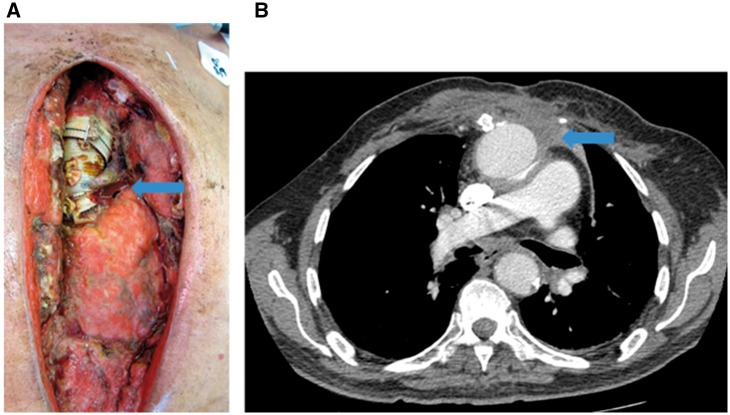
(A) Intraoperative photograph showing aortic graft and *P. aeruginosa* infection (arrow) over myocardium, taken during operation to debride and to wash out infected tissues. (B) Comparative CT image showing infected collection (arrow) and site of targeted aspiration during therapy
